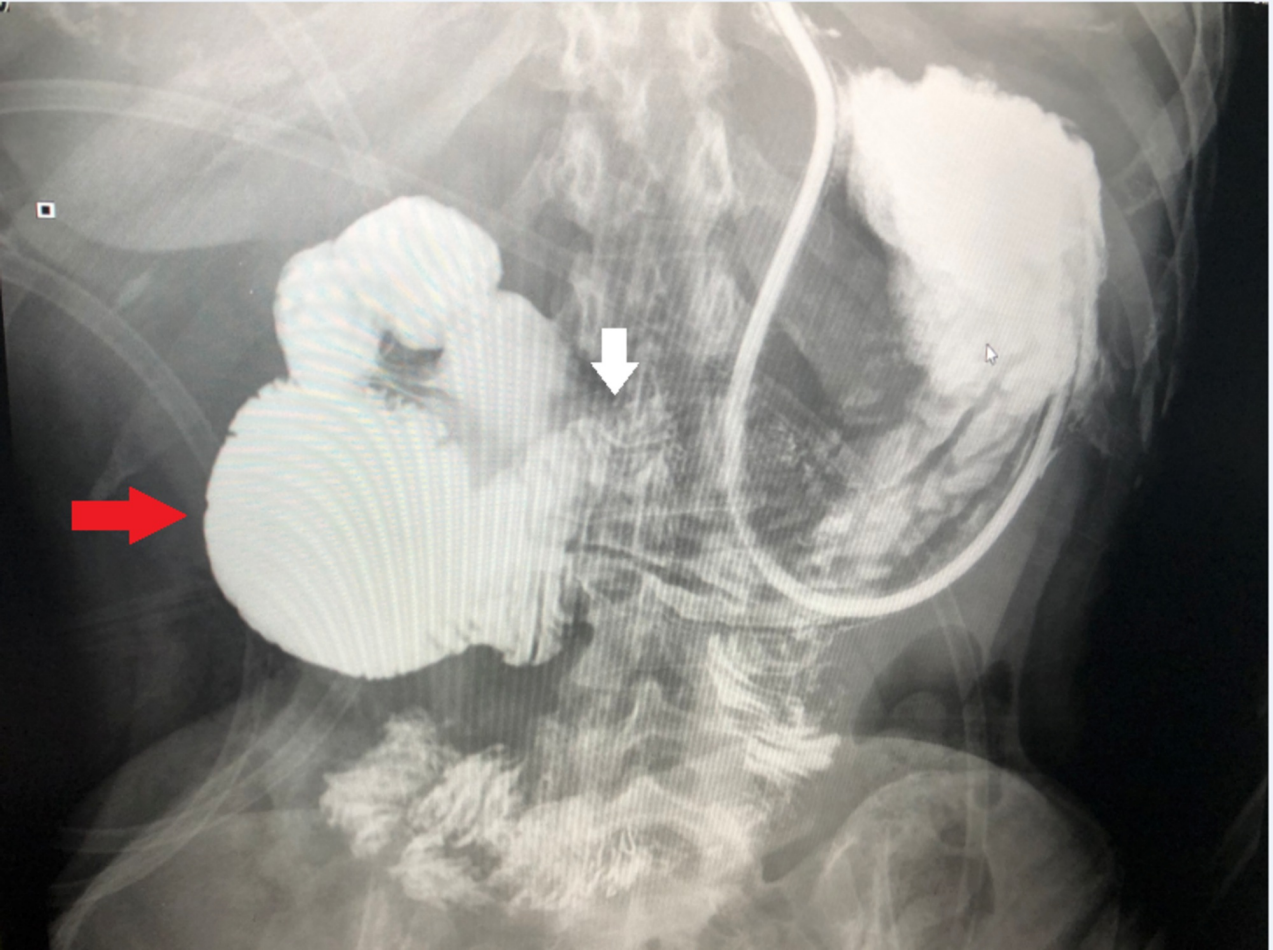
X-ray upper gastrointestinal series with water-soluble contrast showing the transition point; distended duodenum (red arrow) followed by partially obstructed mid-third duodenum (white arrow)
